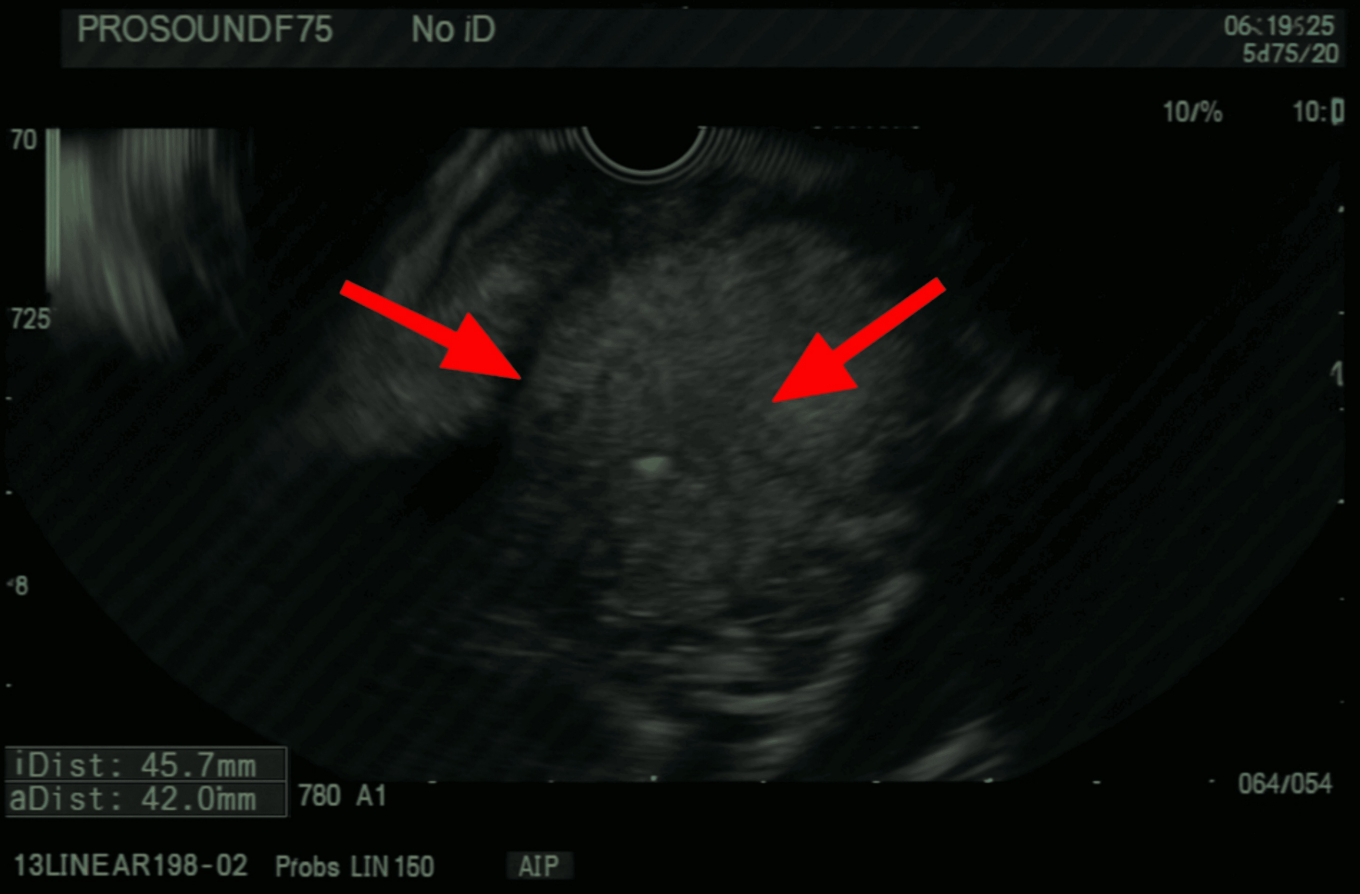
Endosonography showing a malignant-appearing lymph node in the subcarinal mediastinum, measuring 45 mm x 49 mm.
